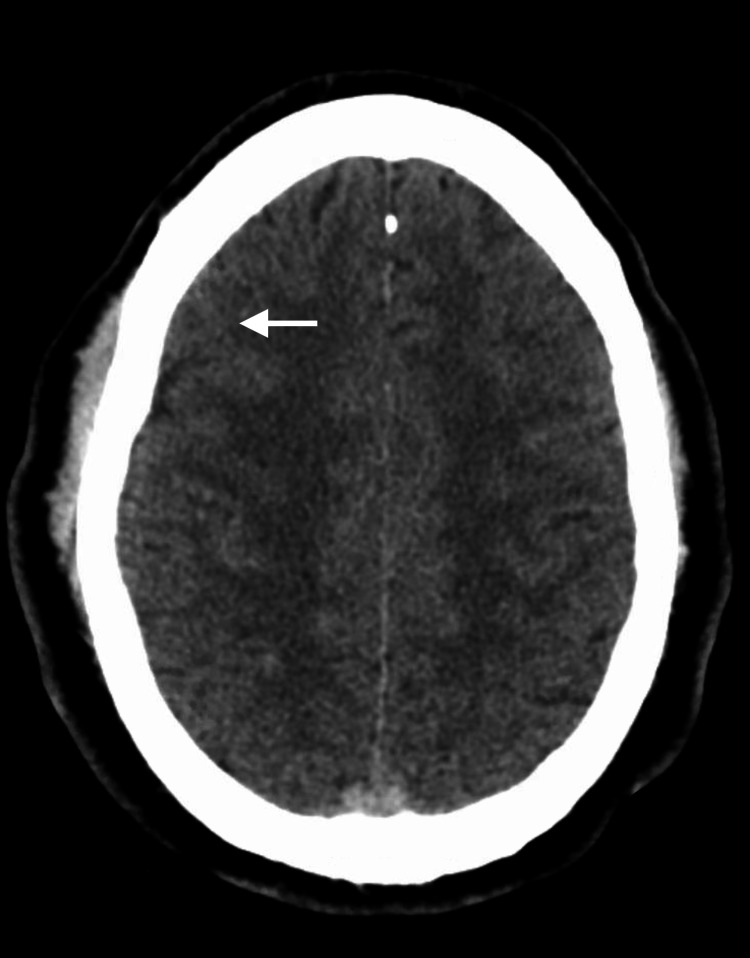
Head CT scan reveals small right parietal subarachnoid hemorrhage. CT: computed tomography
